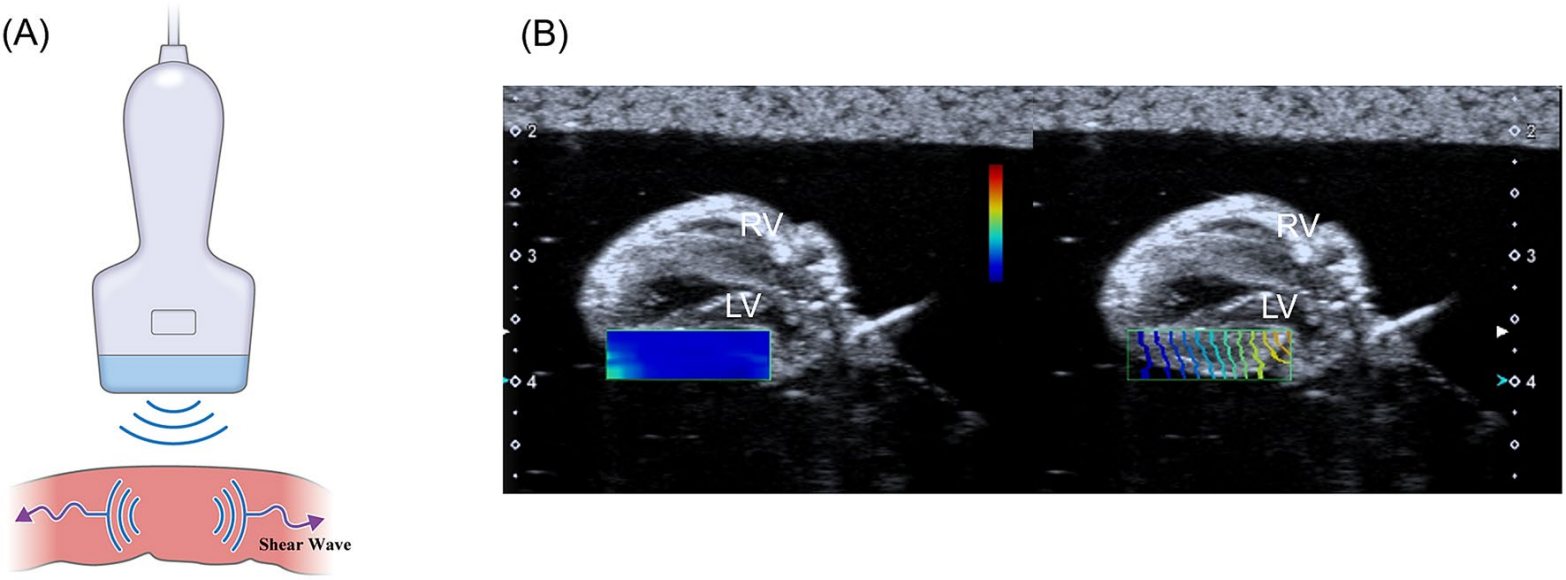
SW imaging. (A) SW was generated by pushing pulse of ultrasound beam. (B) SW propagation on left ventricular free wall. SW elasticity was automatically measured. *LV* left ventricle, *RV* right ventricle, *SW* shear wave.
